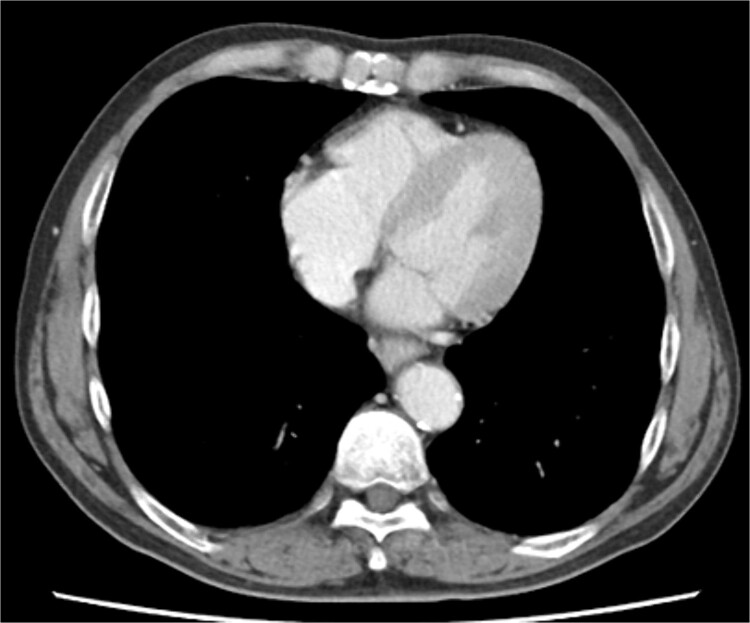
Preoperative contrast-enhanced computed tomography shows no evidence of pericardial effusion, or significant coronary aneurysm.
